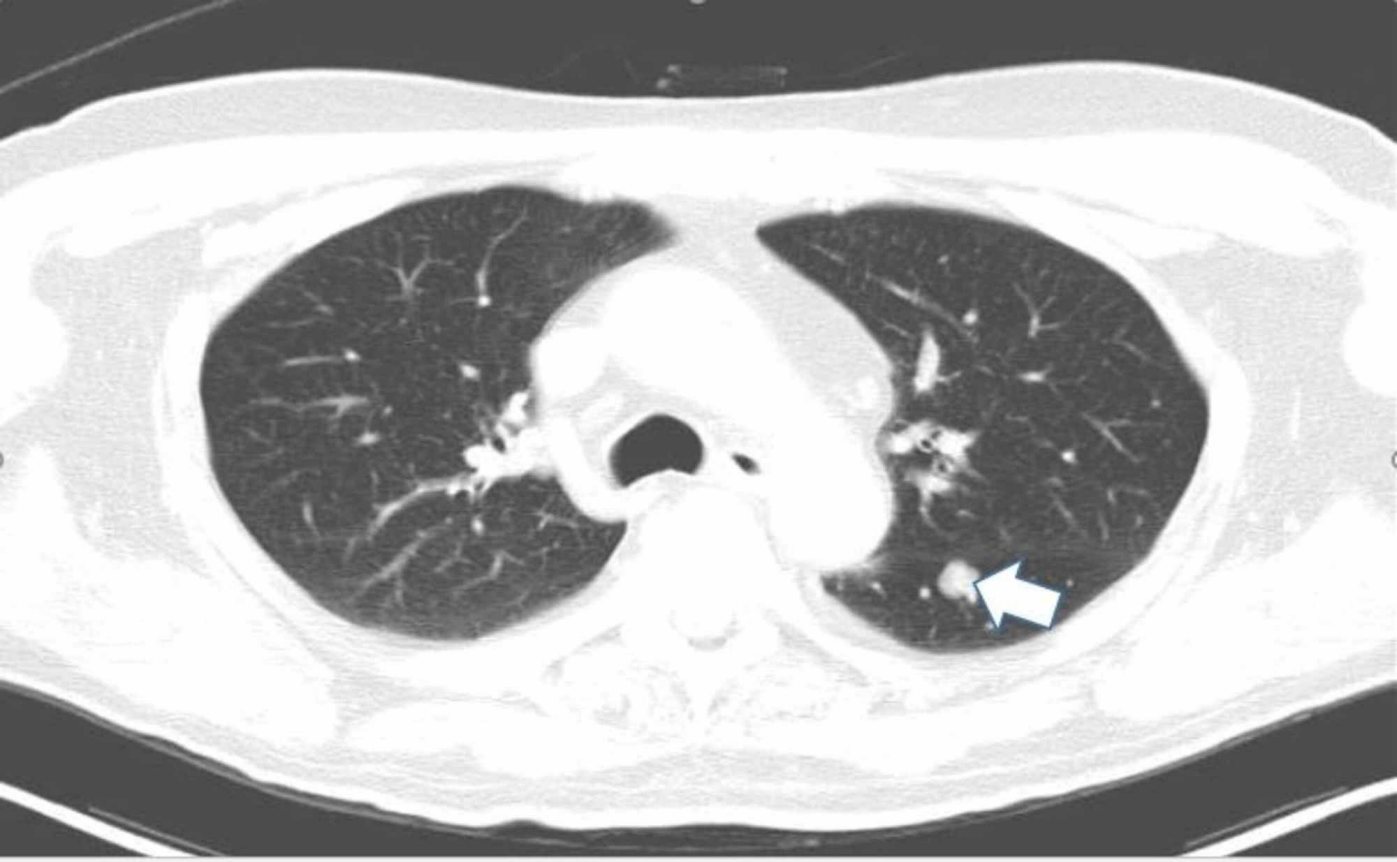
CT scan of the lung in an axial view shows a 10-mm nodule in the left lower lung (white arrow) CT: computed tomography
